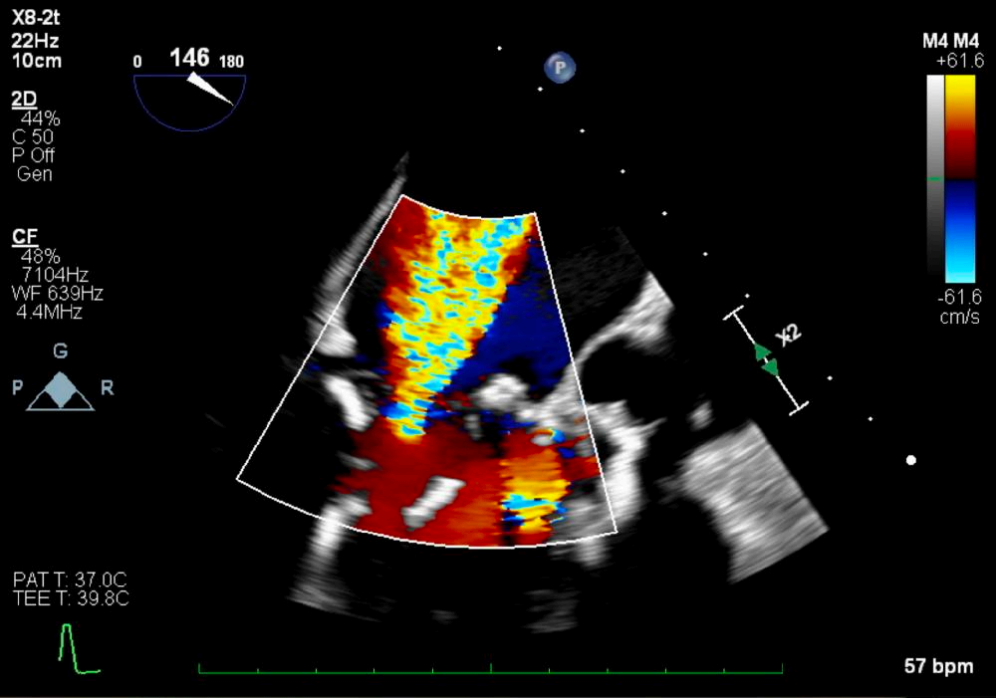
Mitral regurgitation at baseline as assessed by transesophageal echocardiogram.
